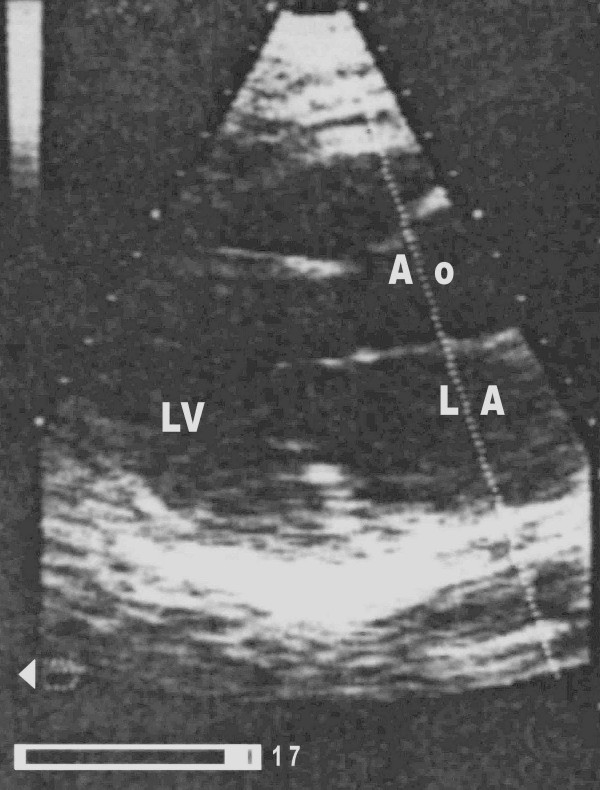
Stress echocardiography: long axis view (baseline). No sign of myxoma in left chamber or in left atrium. LA = left atrium, LV = left ventricle, Ao = Ascending aorta (with kind permission of Dr. Herbst, Potsdam)
